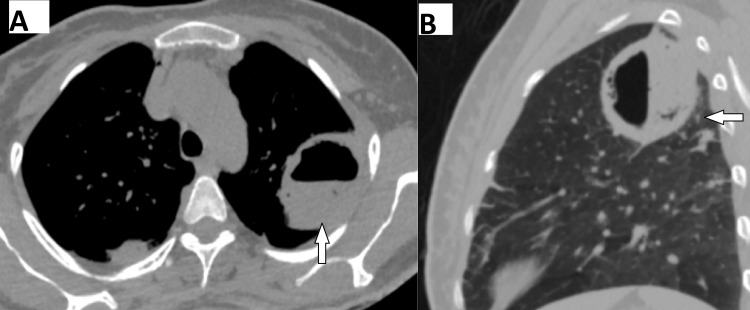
A 48-year-old female with a lung abscess (A) Axial and (B) Sagittal reformatted CT image reveals a thin-walled cavitary lesion with a regular inner margin in the left upper lobe of the lung with an air-fluid level and surrounding ground-glass opacity.
